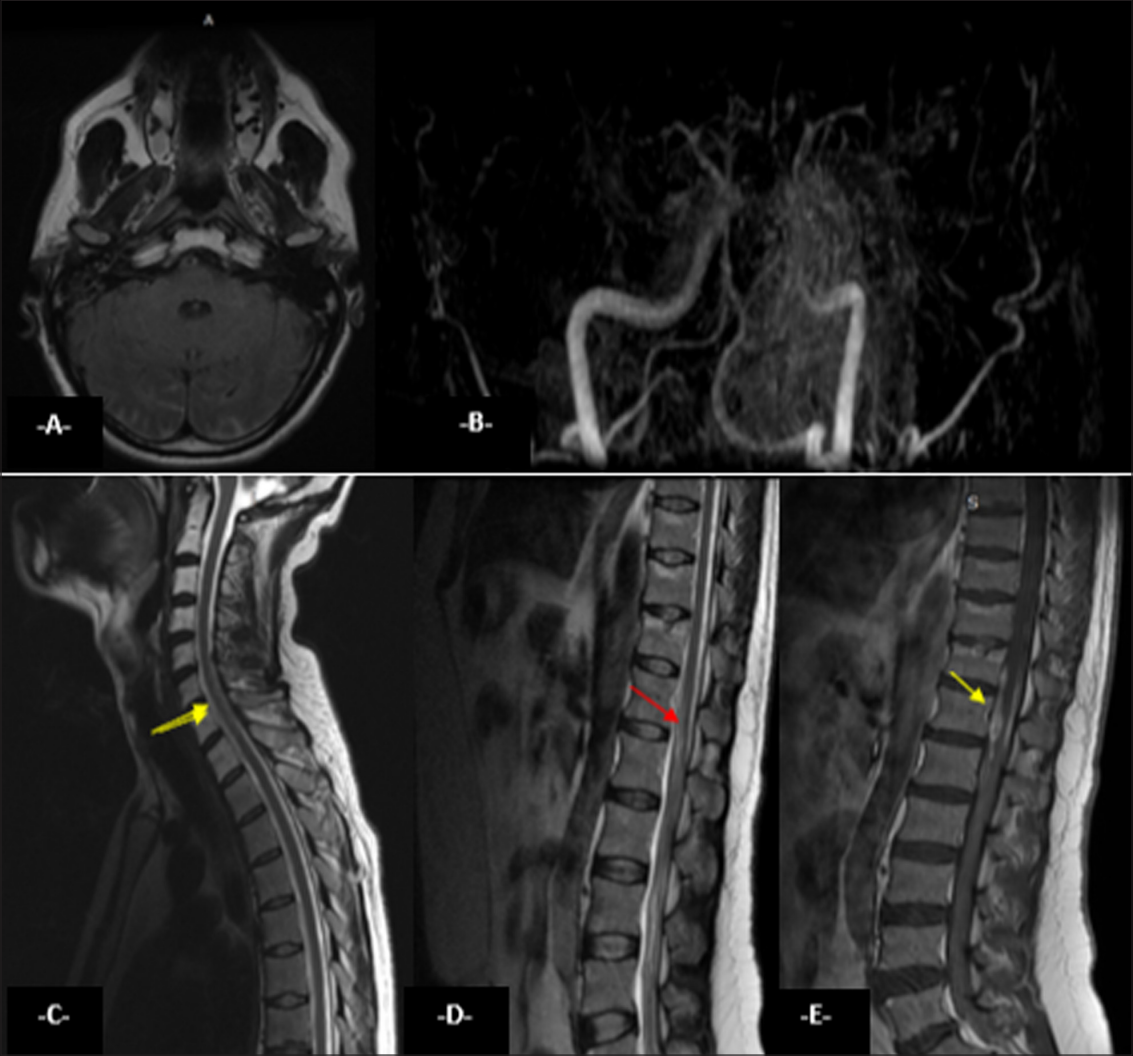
Axial magnetic resonance imaging of the brain showing hyperintensity in the subarachnoid space on T2 FLAIR (A) with a normal Time Of Flight (TOF) angiography. Sagittal magnetic resonance imaging with T2 (C,D) and T1 (E) spin echo (TSE) sequences reveals intradural and epidural hemorrhage (yellow arrows) and hypersignal of the spinal cord from T11 to L1 of the terminal cone (red arrow).
